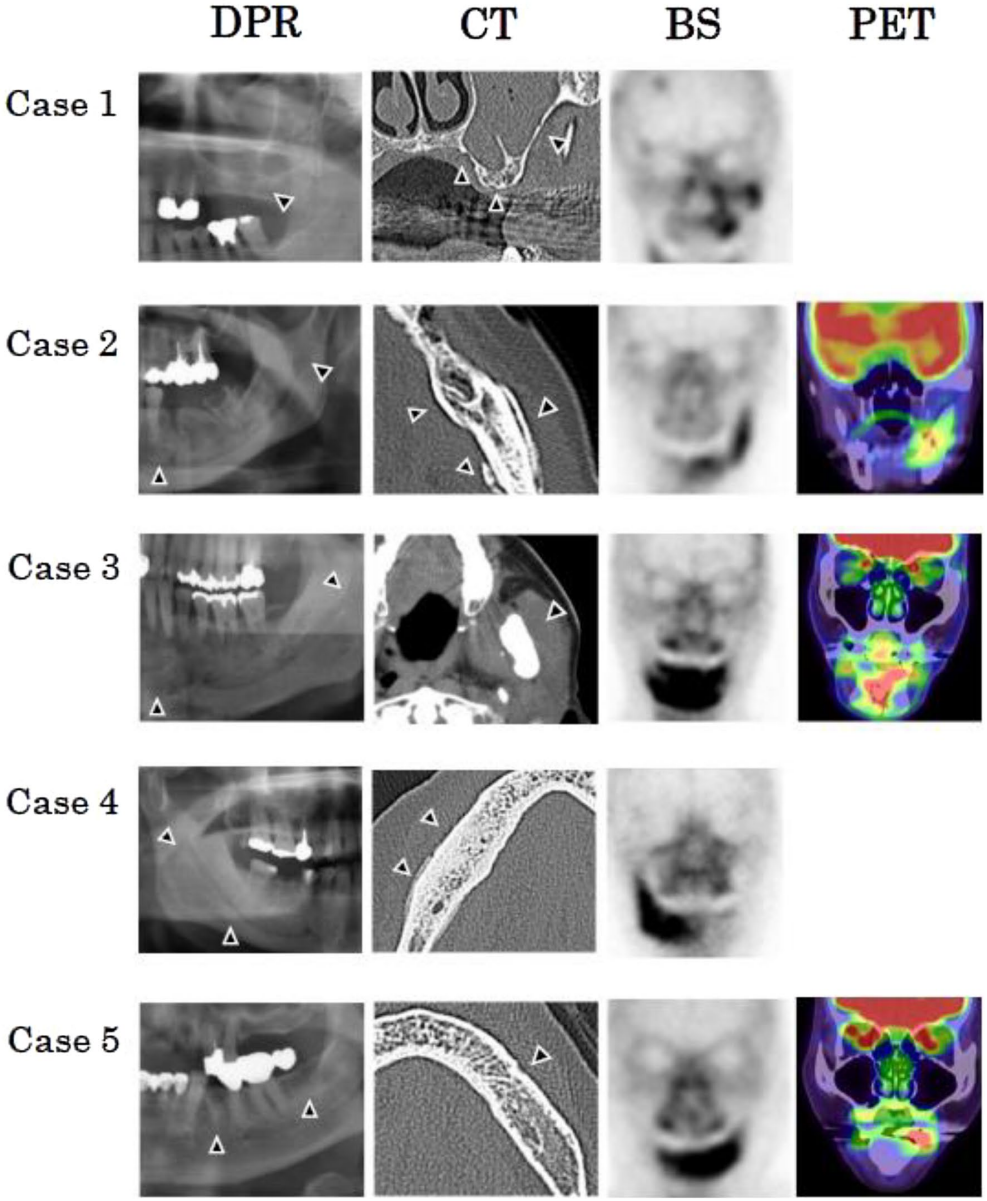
Imaging features in five refractory cases. Patient 1. Location: left molar in the maxilla. a DPR: osteosclerotic changes extending to the maxillary sinus (black arrow); DPR score 1: Grade 2. b CT findings: osteosclerotic changes extending to the maxillary sinus (black arrow); CT score 1: Grade 2. Bone scintigraphy findings: spot accumulation pattern (black arrow). c BS score: Grade 1. Patient 2. Location: left molar region in the mandible. a DPR: osteosclerotic changes extending into the mandibular canal (black arrow). DPR score 1: Grade 2. b CT findings: osteosclerotic changes extending into the mandibular canal and causing a periosteal reaction (black arrow). CT score 1: Grade 2; CT score 2: Grade 1. c Bone scintigraphy findings: spread pattern (black arrow). BS score: Grade 2. FDG-PET/CT: spot pattern. d PET score: Grade 1. SUVmax: 4.41. Patient 3. Location: left molar region in the mandible. a DPR: osteosclerotic changes extending into the mandibular canal (black arrow). DPR score 1: Grade 2. b CT: spread of jaw inflammation to the masticatory muscles (black arrow). CT score 3: Grade 2. c Bone scintigraphy: spread pattern (black arrow). BS score: Grade 2. d FDG-PET/CT: spread pattern. PET score: Grade 2. SUVmax: 6.92. Patient 4. Location: right molar region in the mandible. a DPR: osteosclerotic changes extending into the mandibular canal (black arrow). DPR score 1: Grade 2. b CT findings: osteosclerotic changes extending into the mandibular canal and causing a periosteal reaction (white arrow). CT score 1: Grade 2; CT score 2: Grade 1. c Bone scintigraphy findings: spread pattern. BS score: Grade 2. Patient 5. Location: left premolar region in the mandible. a DPR: osteosclerotic changes in the alveolar bone (black arrow). DPR score 1: Grade 1. b CT findings: osteosclerotic changes in the alveolar bone (white arrow). CT score 1: Grade 1. c Bone scintigraphy findings: spread pattern. BS score: Grade 2. d FDG-PET/CT: spot pattern. PET score: Grade 1. SUVmax: 8.75. *BS* bone scintigraphy, *DPR* dental panoramic radiography, *CT* computed tomography, *FDG* 2-(18F)-fluoro-2-deoxy-D-glucose, *PET* position emission tomography, *SUV*_*max*_ maximum standardized uptake value
